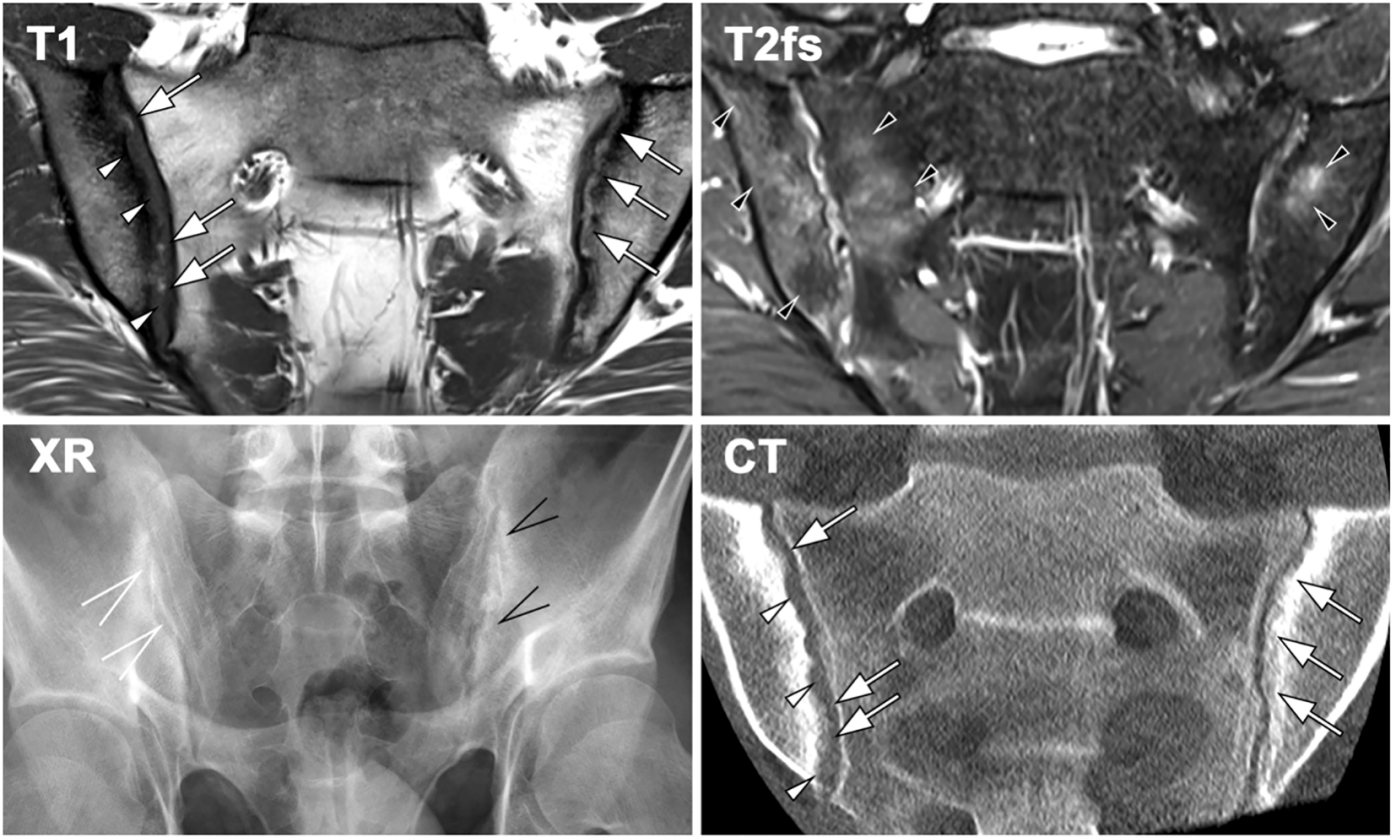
Imaging findings in a 23-year-old patient with sacroiliitis in axial spondyloarthritis (axSpA), demonstrating characteristic features across different modalities. MRI-T1 reveals erosions (white arrowheads) and new bone formation in the form of backfill (white arrows), accompanied by sclerosis. Fat-suppressed T2-weighted MRI detects bilateral bone marrow oedema (BMO, black arrowheads). Radiography (XR) shows erosion and new bone formation on the left sacroiliac joint (SIJ, black open arrows), whereas the right SIJ exhibits only minimal changes, including joint space widening (white open arrows). CT provides comparable visualization of joint erosion (white arrowheads) and new bone formation (white arrows), consistent with MRI-T1 findings.
